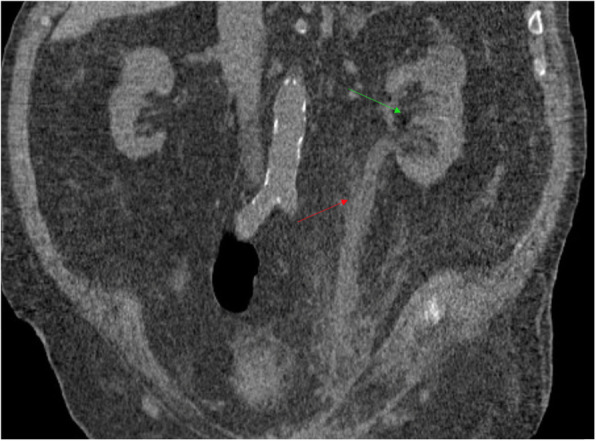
CT scan of the abdomen and pelvis post-operation day 20 showing improvement in hydroureternephrosis. (Green arrow: Hydronephrosis, Red arrow: ureteronephrosis)
